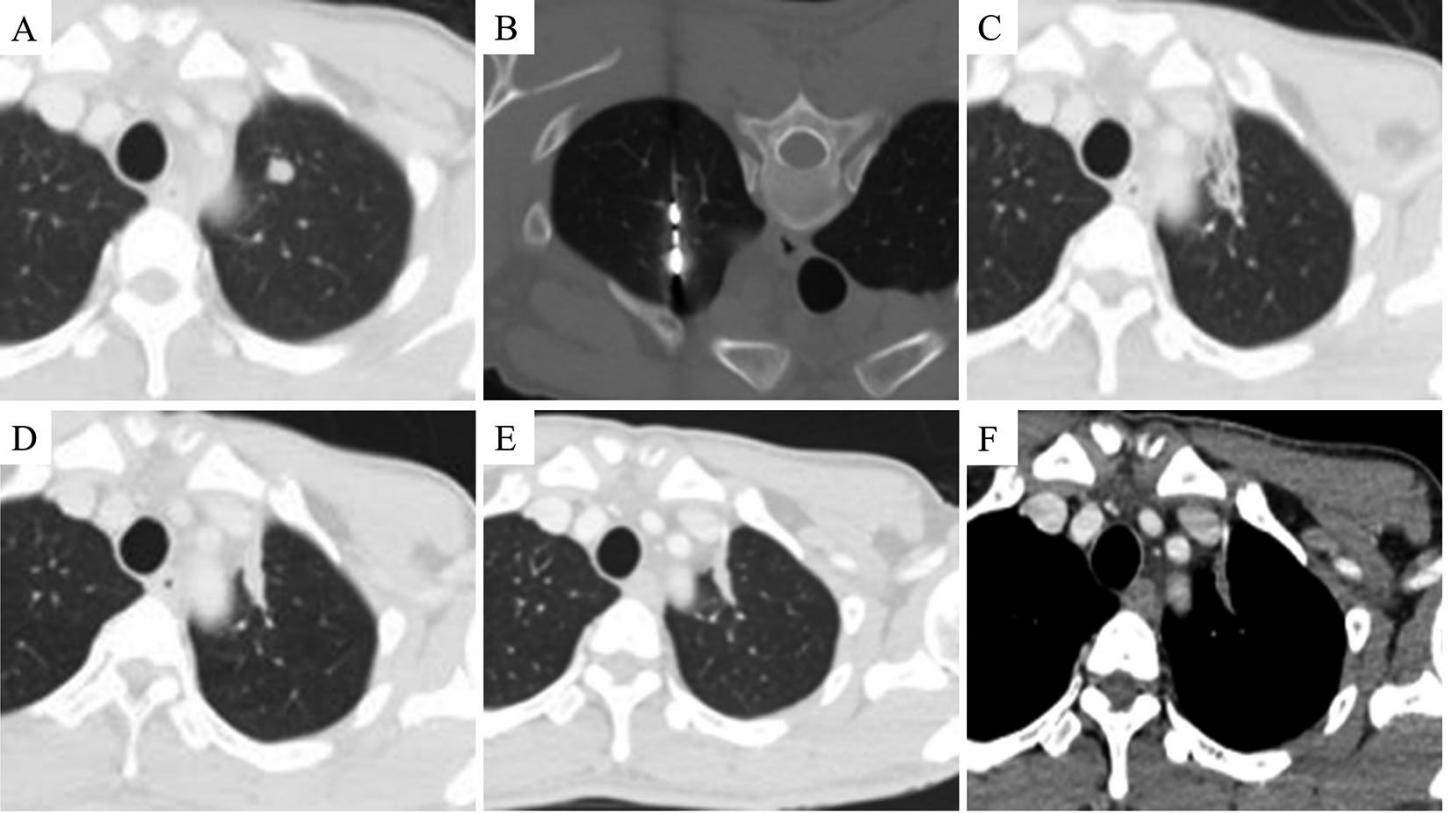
A 48-year-old male had a nodule in the upper left lung lobe for over four months, 16 months after primary hepatoma carcinoma surgery. A A 1.3 × 1.3 cm round nodule was seen in the left upper lung. B With the patient in the prone position and under local anesthesia with 2% lidocaine, CT-guided puncture MWA was performed. C A month after MWA, the lesion lost its original shape, and the surrounding exudate declined. D Six months after MWA, the lesion size was further reduced. E, F A year after MWA, the lesion shrank to a fibrous cord with no enhancement. The efficacy was assessed to achieve complete ablation
